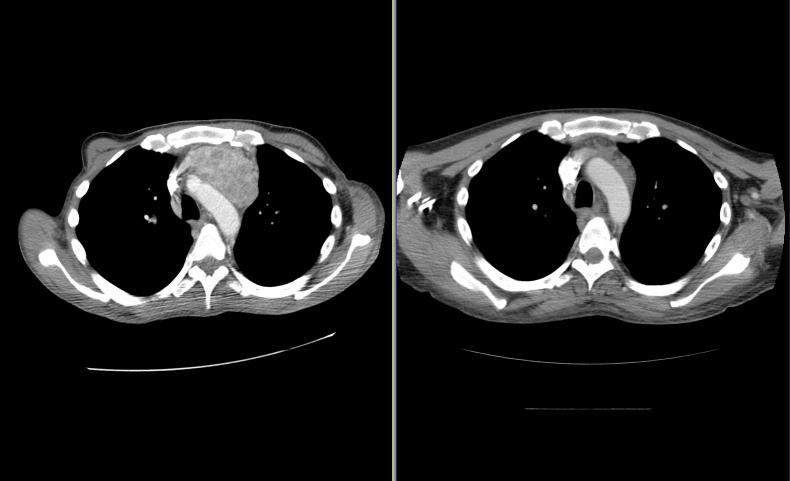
CT slice showing resolution of paratracheal/paraesophageal mass after image-guided SBRT. Left image is pre-treatment while right is post-treatment.
